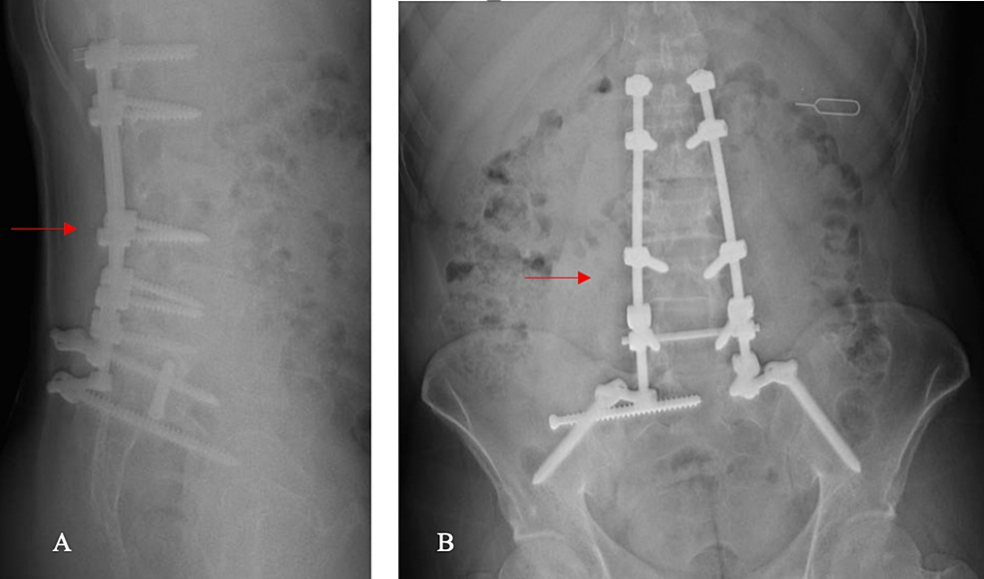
X-rays at one-year follow-up A: Lateral; B: Anteroposterior (AP). The arrows show stable fixation at follow-up.
